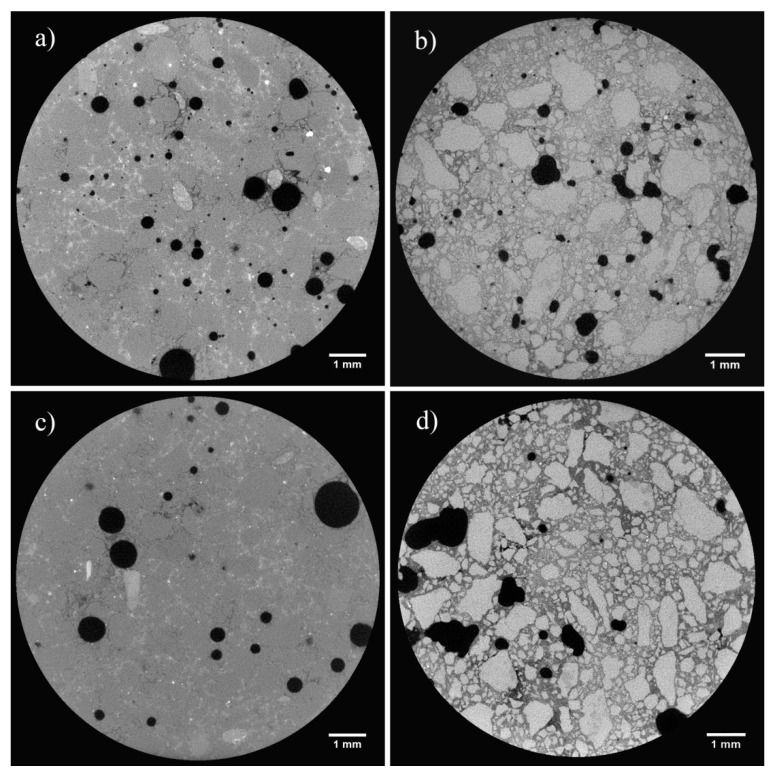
Slices of X-ray tomography of samples (a) CIQ, (b) CIM, (c) CIVQ and (d) CIVM.
